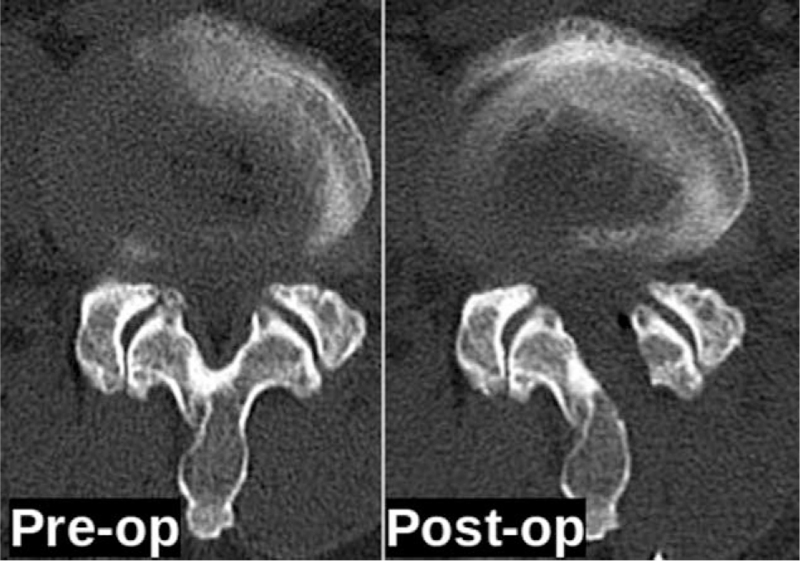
The CT scan axial cut of the same patient at preoperative and immediate postoperative point of time shows the approach from left side with preservation of ipsilateral and contralateral facets. CT = computed tomography.
